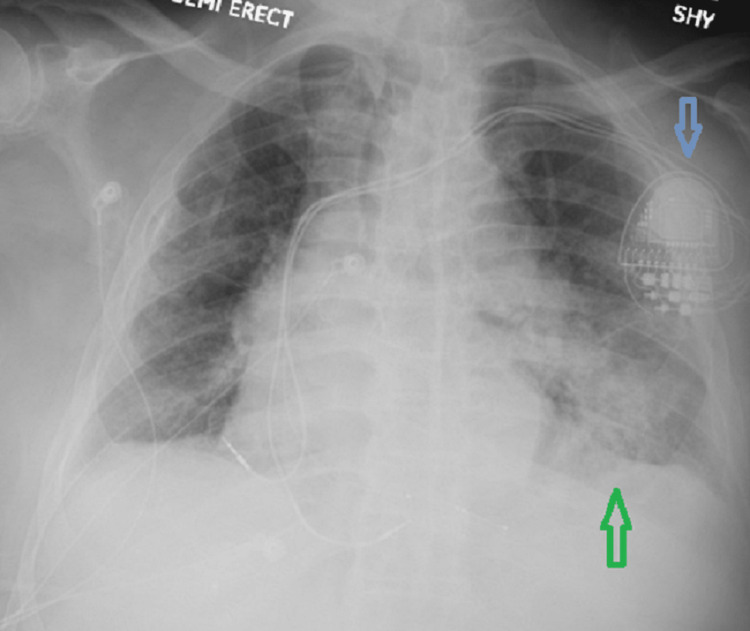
Anterior posterior view of portable chest x-ray (CXR) with blue arrow showing pacemaker and green arrow pointing toward the left lower lobe infiltrates
